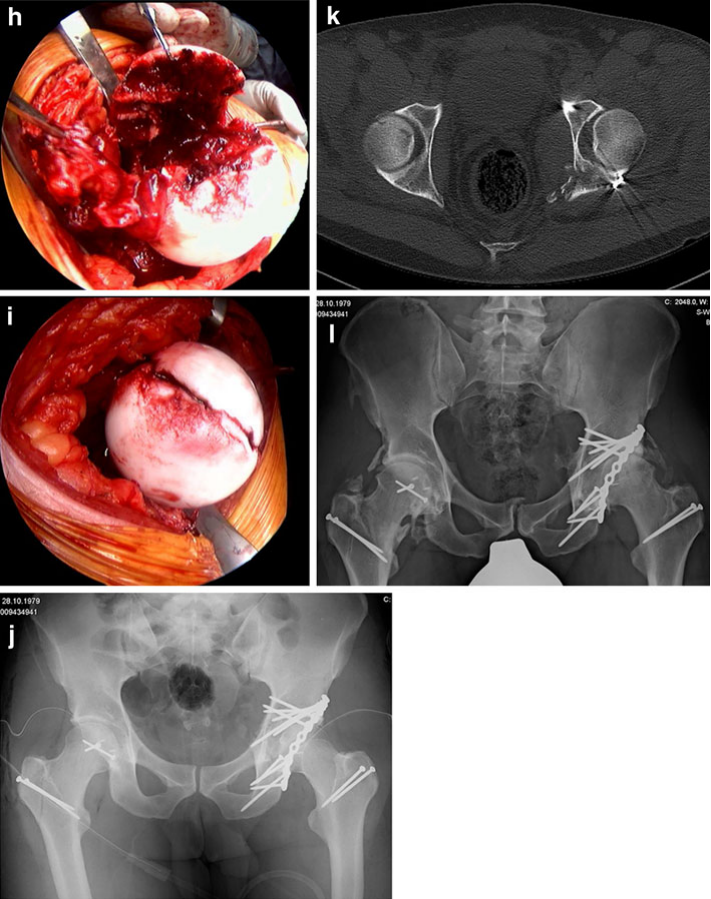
a Anteroposterior radiograph of the pelvis of a 30-year-old patient who sustained bilateral posterior hip dislocations due to a work accident before closed reduction. b Anteroposterior radiograph of the pelvis after closed reduction. c Axial CT scan shows a transverse acetabular fracture with comminuted posterior wall fracture of the left hip. d Coronal CT scan demonstrates a femoral head fracture (Pipkin type I) on the right side. e Intraoperative view of the left acetabulum through the posterior approach shows marginal impaction and comminuted posterior wall fracture. f Trochanteric flip osteotomy and surgical hip dislocation allows complete exposure of the transverse fracture with comminuted posterior wall fracture. g Exposure of the acetabulum after anatomic reduction and internal fixation. h Intraoperative view of the right femoral head before anatomic reduction and fixation after surgical hip dislocation. i Intraoperative view of the right femoral head after anatomic reduction and fixation. j Postoperative anteroposterior radiograph of the pelvis demonstrates anatomic reconstructions of the Pipkin I fracture on the *right side* and of the acetabular fracture on the *left side*. k Postoperative CT scan demonstrates anatomic reconstructions of the Pipkin I fracture on the *right side* and of the acetabular fracture on the left side. l Anteroposterior radiograph of the pelvis 3 years after surgery shows bilateral ectopic ossifications (Brooker grade 2) and moderate signs of posttraumatic osteoarthritis of the left hip of the patient with an excellent clinical result
